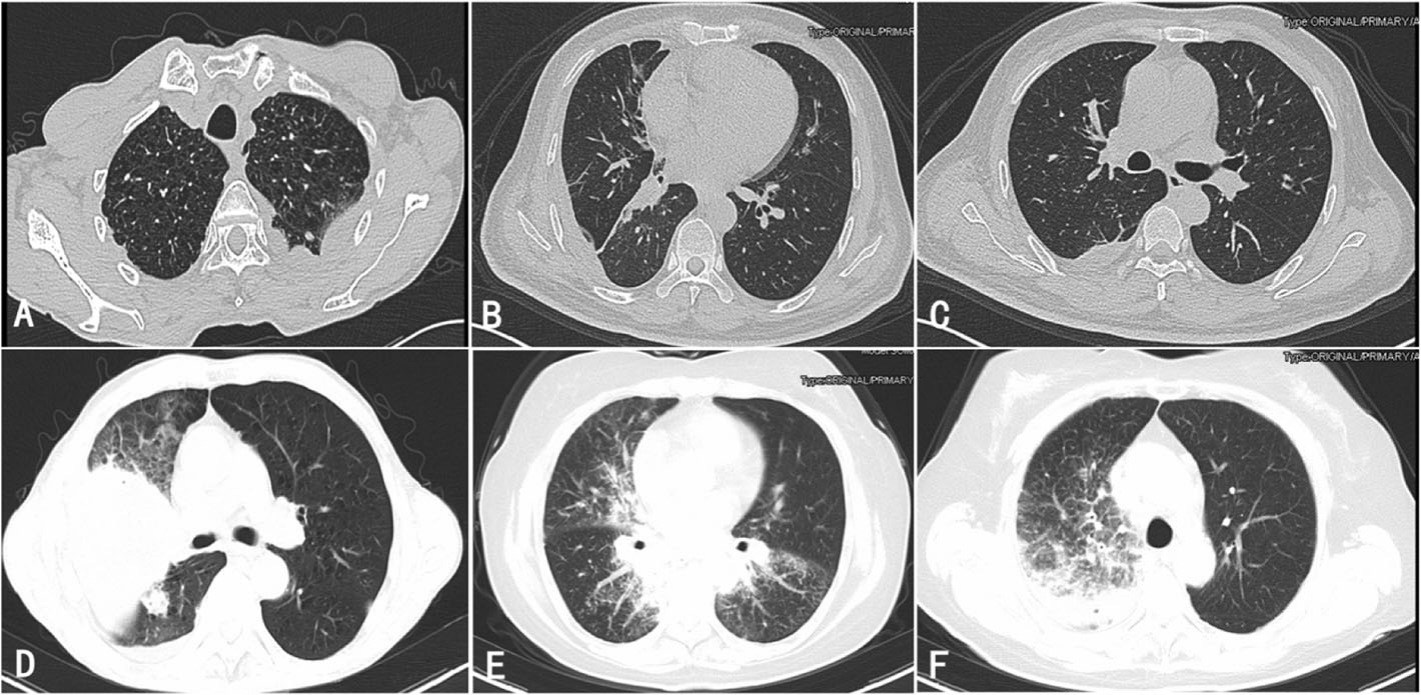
Other radiological manifestations of lungs in IgG-RD patients via chest computed tomography scans. a Thickening of the left pleura can be observed. A small nodule near the thickened pleura can also be noted. b/c Thickening of interlobular septa and pleura effusion in the right lung can be observed. d Mass and ground-glass opacity were shown. e/f Small lung nodules, combined with ground-glass opacity, consolidation, thickening of interlobular septa, and thickening of bronchial wall, can be observed
